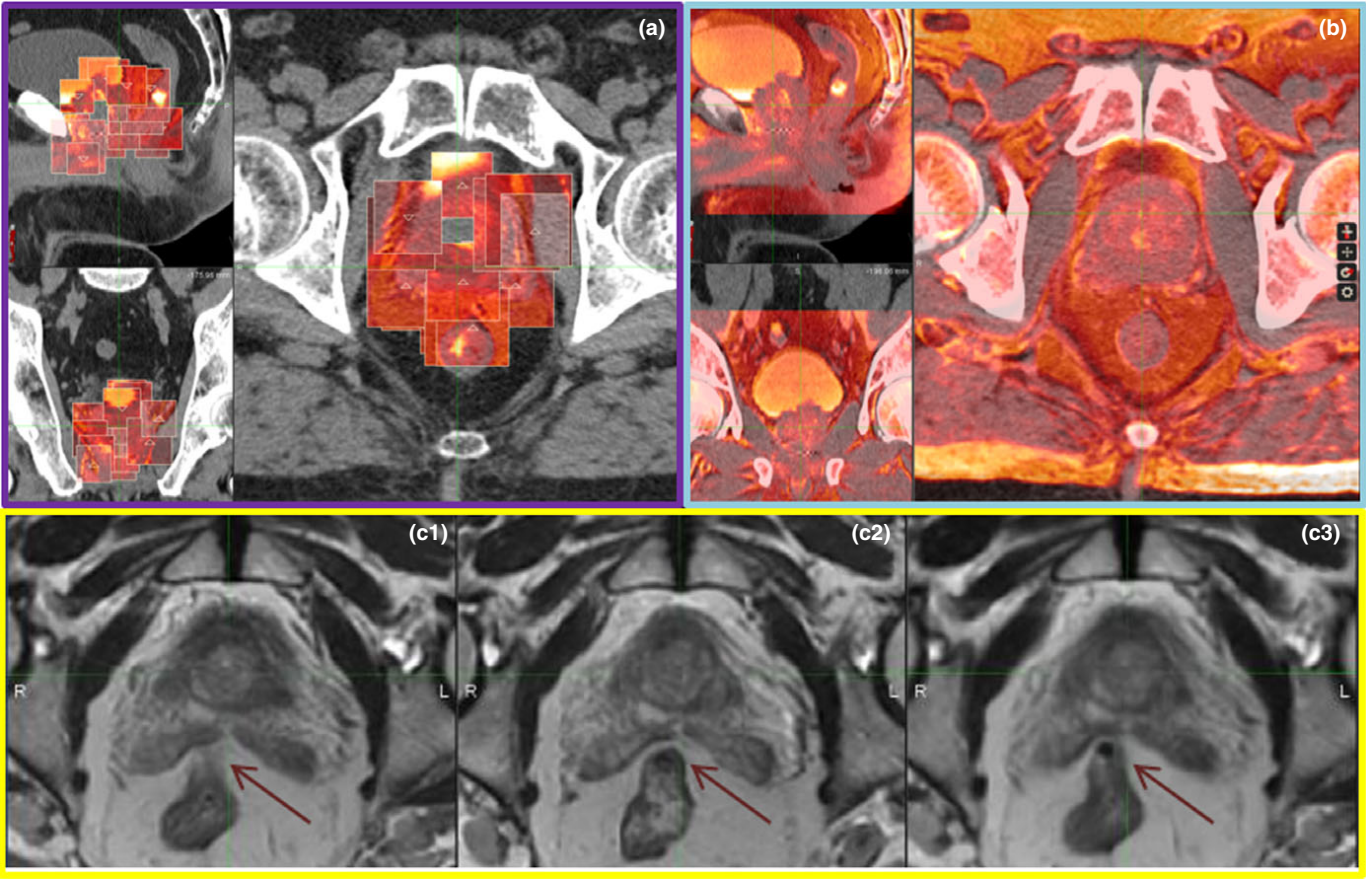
DIR procedure. (a) Local alignments used by the DIR (sagittal, coronal, and axial). MRI alignments are overlaid in red on top of the planning‐CT; (b) Resulting deformed MRI dataset blended with the planning‐CT; (c) diagnostic‐MRI(c1), planning‐MRI(c2) and deformed diagnostic‐MRI(c3). Note how the rectum in the deformed diagnostic‐MRI(c3) correlates well with the planning‐MRI(c2).
